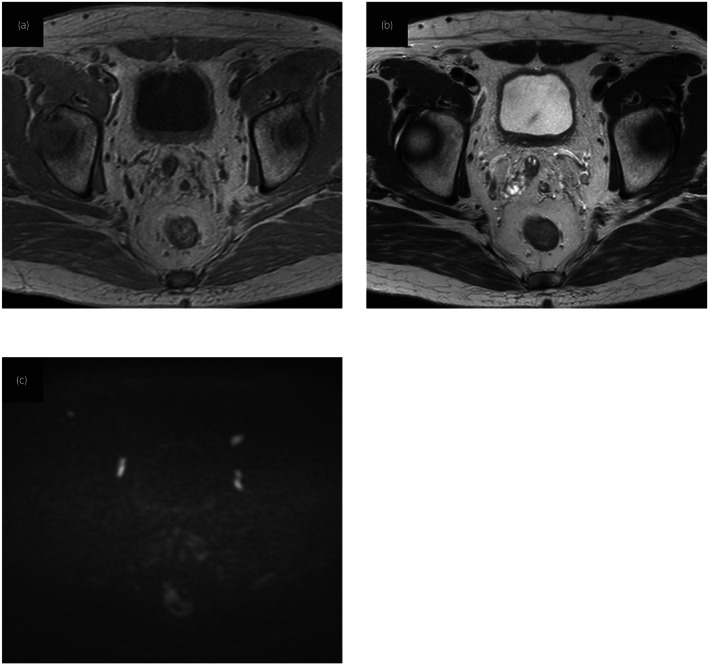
Magnetic resonance imaging after BCG therapy showing improvement. The bladder wall displayed homogeneous hyperintensity relative to the muscle on T1WI (a), hypointensity on T2WI (b), and diffusion was hardly restricted on DWI (c).
